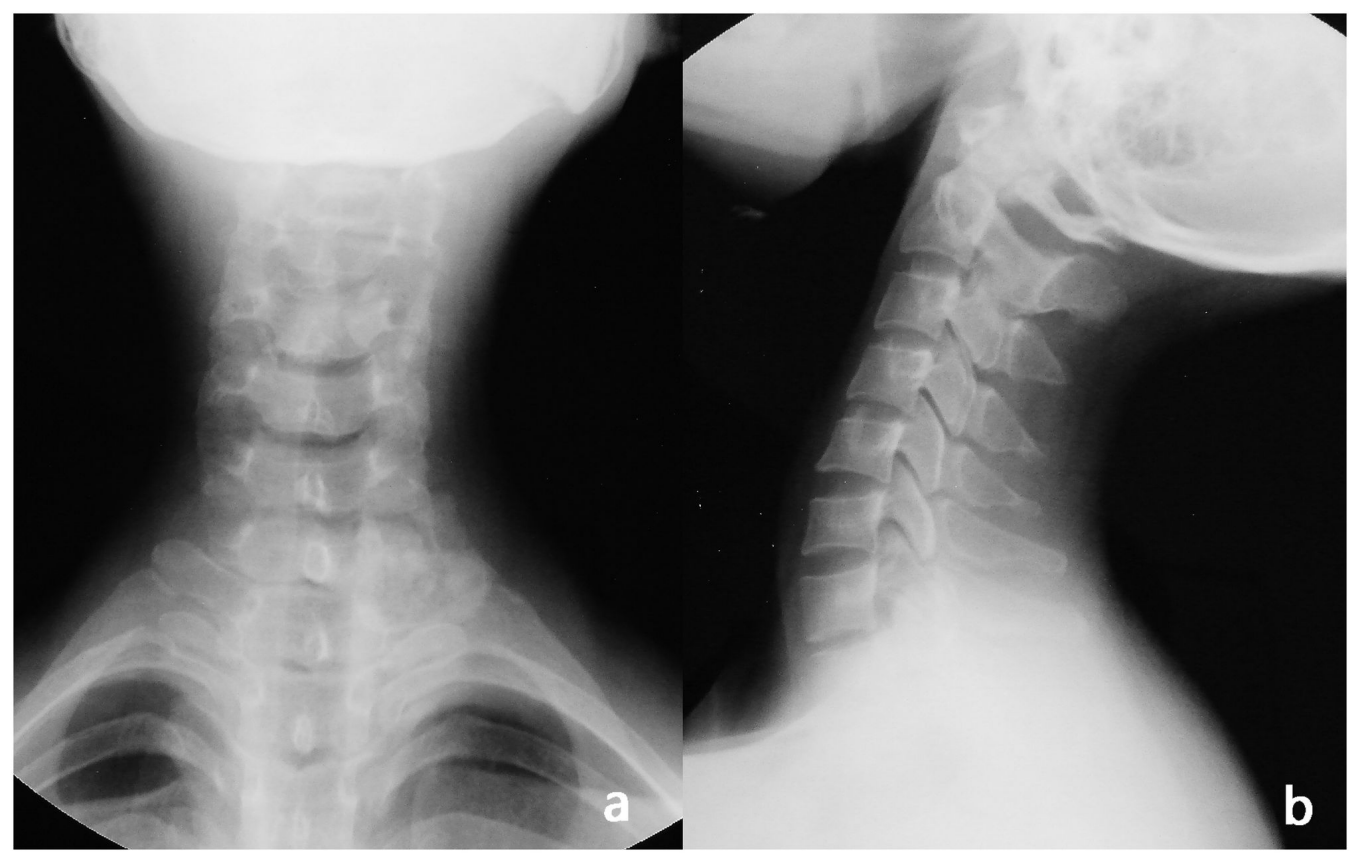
Anteroposterior and lateral radiographs of the cervical vertebrae of a 33year-old female. a: anteroposterior radiograph; b: lateral radiograph. The patient was admitted to our hospital with primary complaints of neck and back pain for 4 years, with pain radiating to the right upper arm and numbness in right hand for 3 years.
